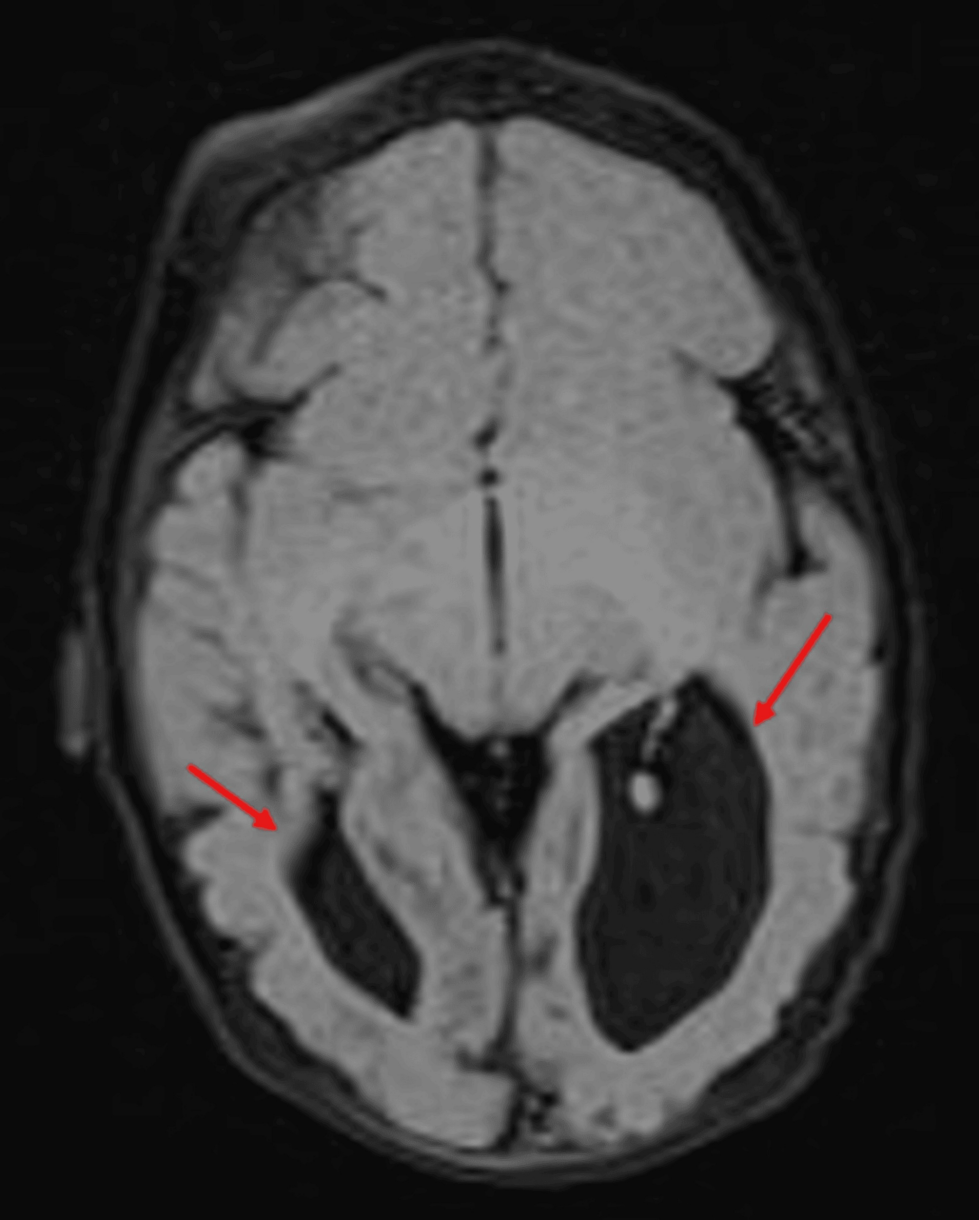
T2 FLAIR axial view brain MRI showing bilateral cerebral dysgenesis at the occipital head region (arrows). FLAIR: fluid-attenuated inversion recovery, MRI: magnetic resonance imaging
